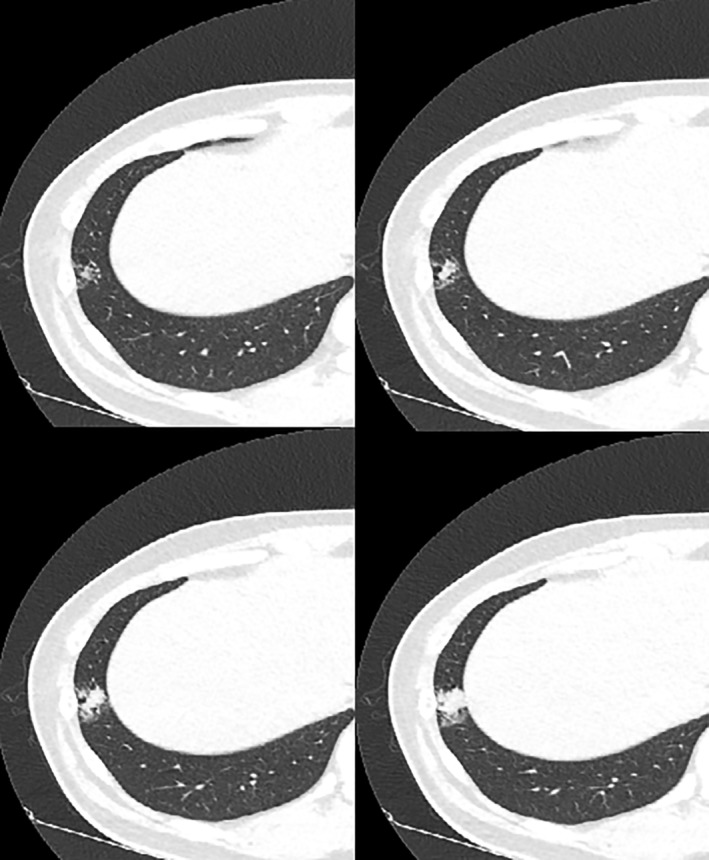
A preoperative chest computed tomography scan shows a focal consolidative lesion with bronchiectasis in the subpleural region of the lateral basal segment of the right lower lobe.
